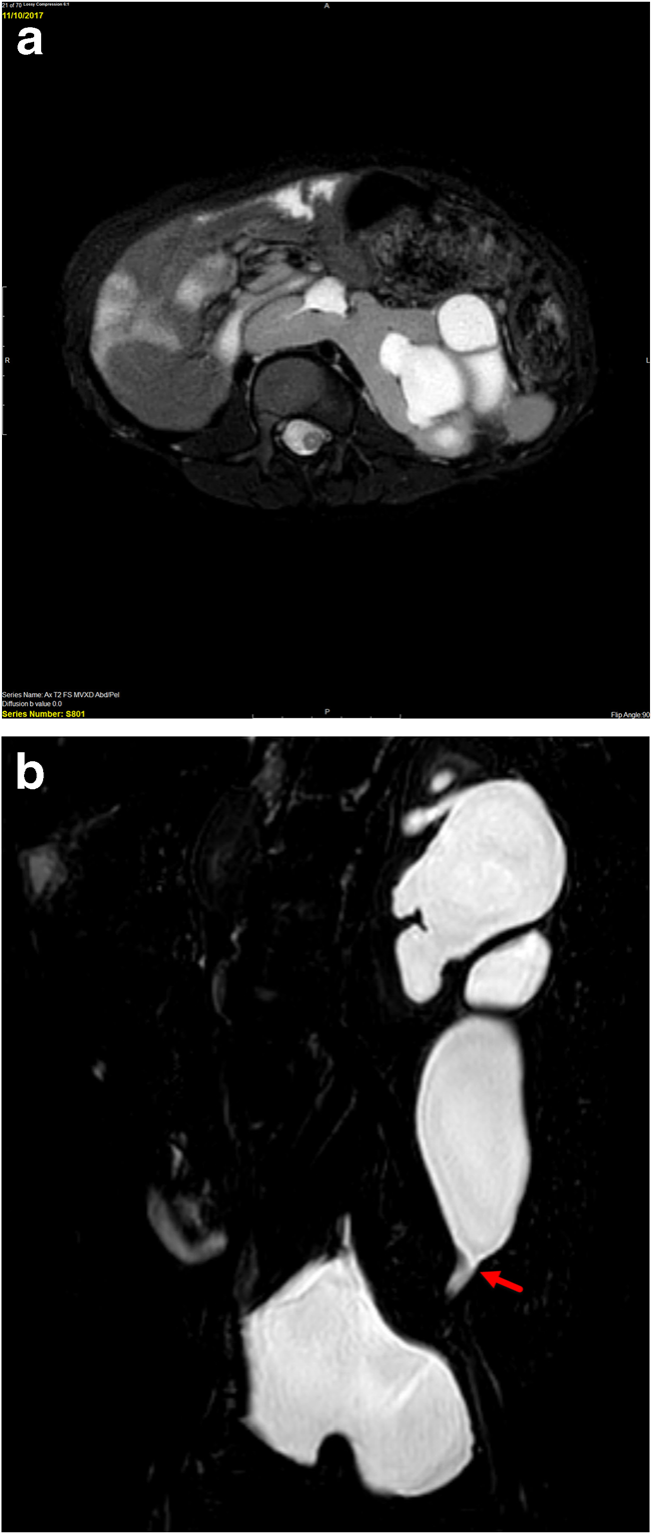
Four-year-old with history of horseshoe kidney who has previously undergone left pyeloplasty but has persistent hydronephrosis and ureteral dilation and clinical concern for obstruction. a Axial T2-weighted fat suppressed image shows renal parenchyma crossing the midline anterior to the spine (blue arrow) consistent with a horseshoe kidney. There is moderate pelvocaliectasis of the left moiety (red arrow). b Coronal T2-weighted image shows abrupt narrowing of the distal left ureter (red arrow). At surgery, the stricture was found to be related to scar tissue from prior pyeloplasty
